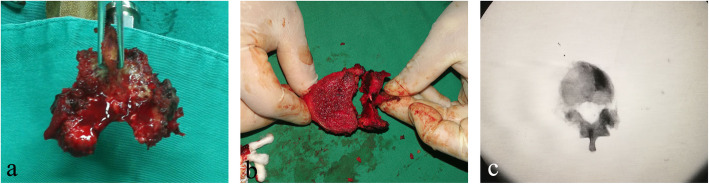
TES technique. a En bloc dorsal elements. b En bloc resection of the ventral vertebral body. c Radiograph showing the complete removal of the diseased vertebra
